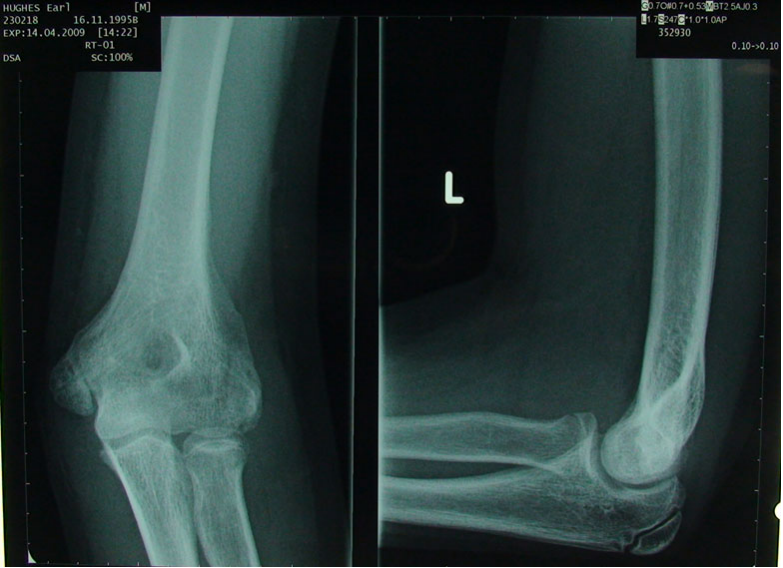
Final follow-up X-ray (showing fully united medial and lateral epicondyles).
